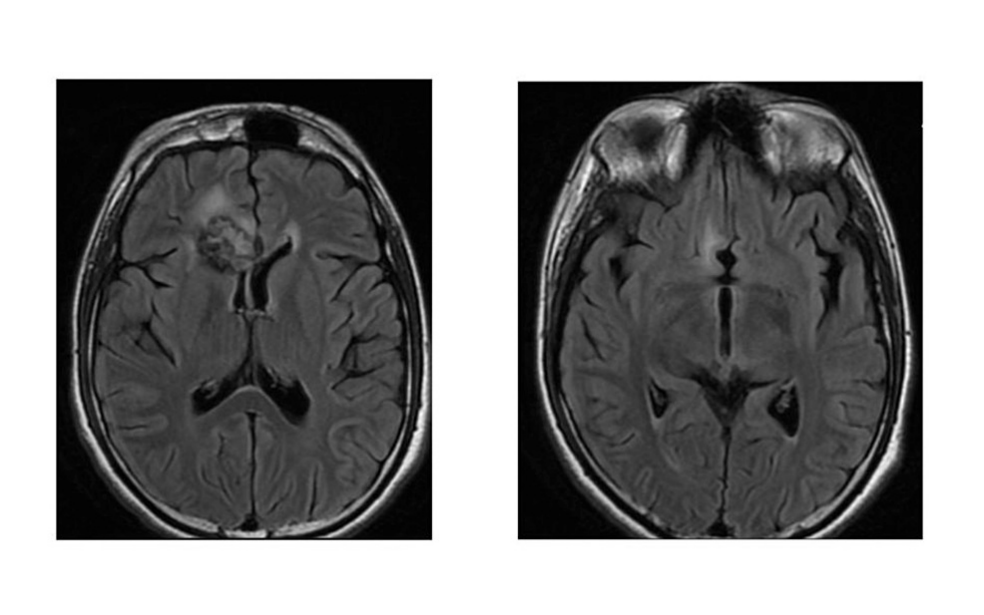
Post-treatment MRI imaging showing the response of the lesion
